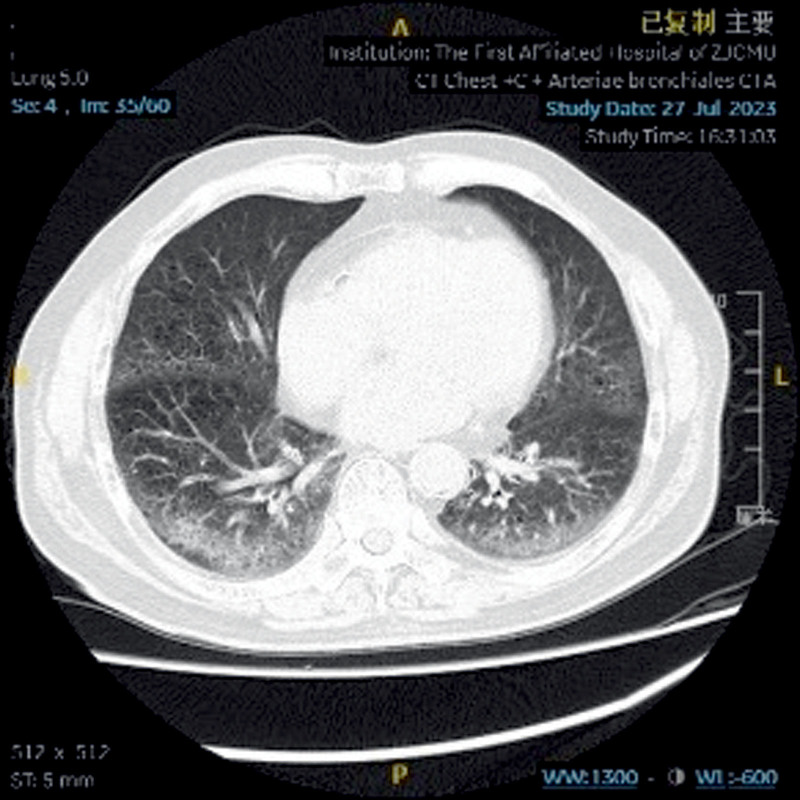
Plain chest computed tomography (CT) scan indicated pulmonary emphysema in both lungs and interstitial inflammation in the lower lobes of both lungs.
